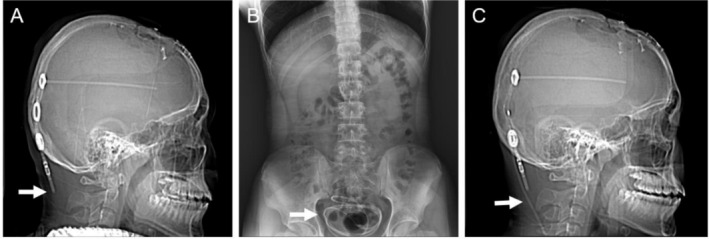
(A) CT topogram demonstrated an abrupt interruption of the occipital VP catheter with no visible drainage tube, confirming VP shunt disconnection (white arrow). (B) Abdominal X‐ray showed the VP catheter coiled in the pelvis, completely detached from its connection site (white arrow). (C) CT topogram demonstrated reconnection of the VP catheter after revision surgery (white arrow).
